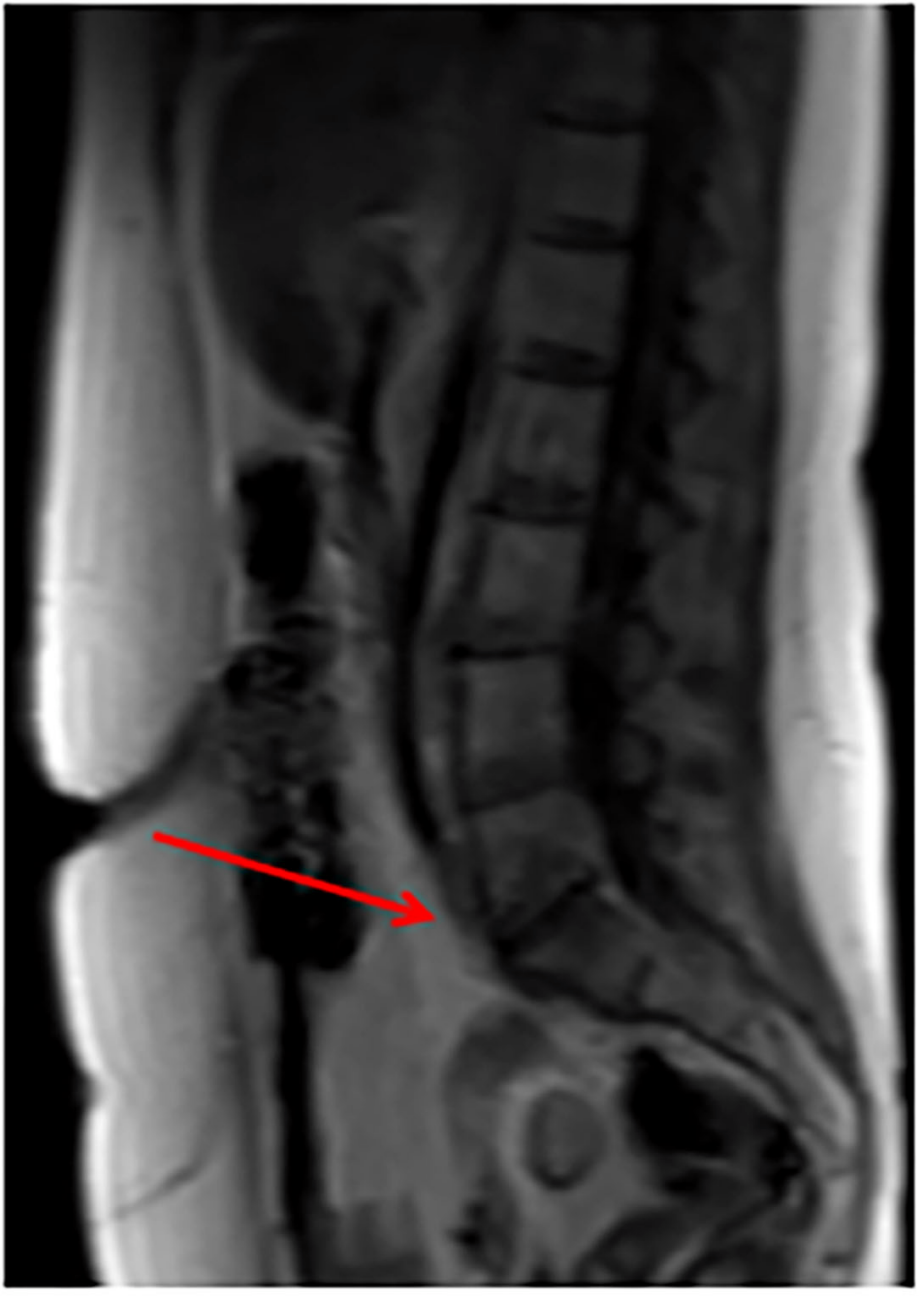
Pelvic MRI 169 days after the second surgery showing a progressive decrease of the purulent collection and a spontaneous resolution of the fistula.
